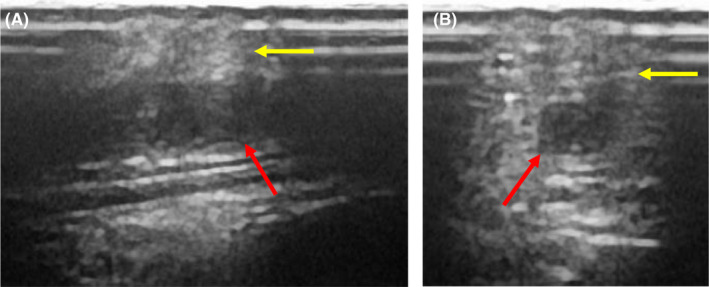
Vascular ultrasonography showed a thrombus in the lumen of the cephalic vein of the left forearm (red arrow), accompanied by inflammatory findings in adjacent tissues (yellow arrow). (A) Longitudinal view. (B) Transverse view
